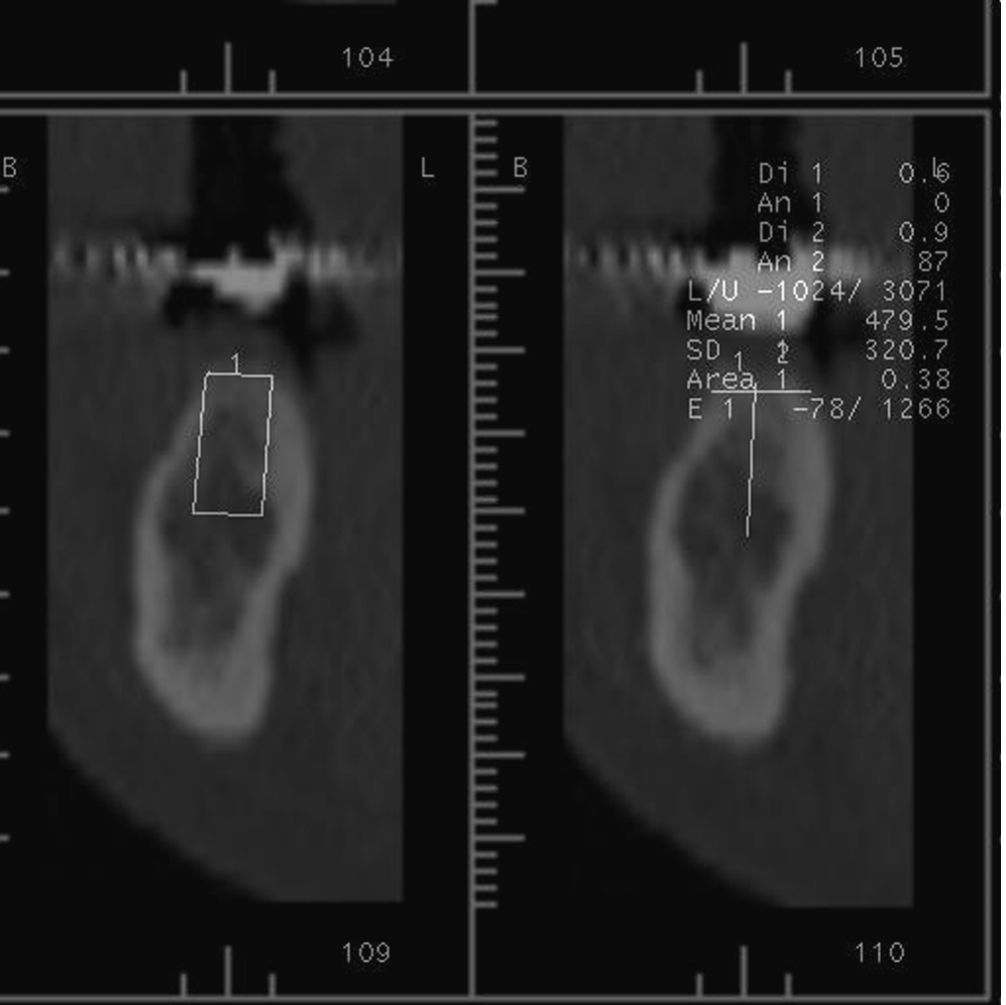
Cross-sectional CT image of the implant recipient site evaluated in this study.
